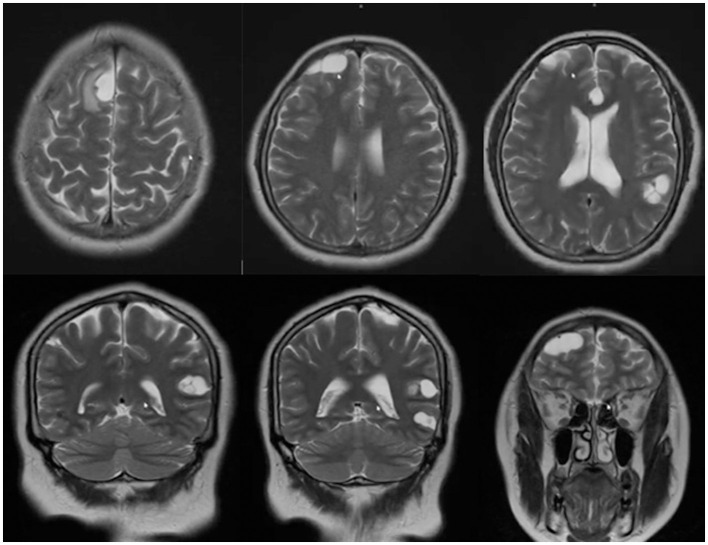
Non-contrast brain MRI – axial FLAIR sequence.
